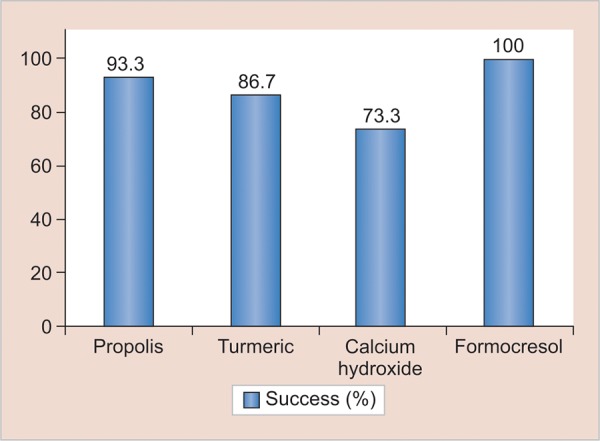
Success percentage assessed by radiographic findings
